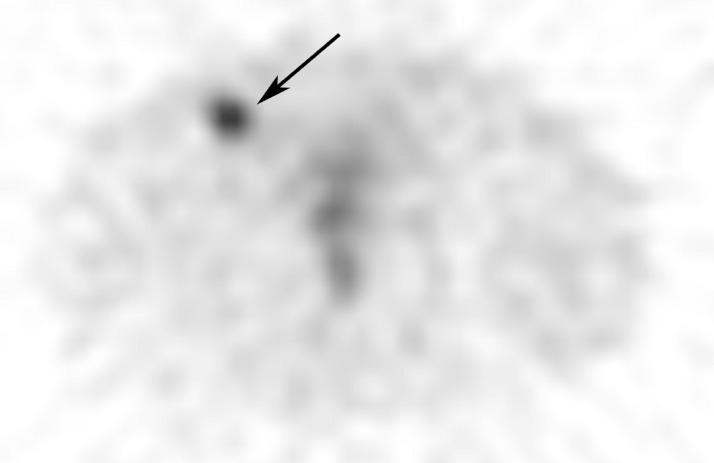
Octreotide Scintigraphy with Single-Photon Emission Computed Tomography (SPECT) An axial image obtained from single-photon emission computed tomography (SPECT) acquisition through the pelvis 24 hours following the IV injection of 5.9 mCi Indium-111 pentetreotide (OctreoScan, Mallinckrodt Pharmaceuticals, Maryland Heights, MO), a somatostatin analogue. An abnormal increased radiotracer uptake was identified (black arrow) in the right anterior lower pelvis corresponding to the enlarged right inguinal lymph node seen on the CT scan. This finding indicates nodal metastasis from the patient’s previously resected small bowel neuroendocrine tumor.
